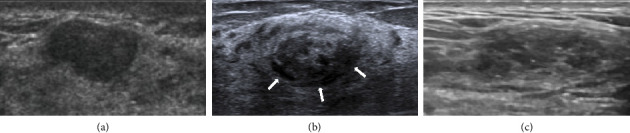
(a) Ultrasound presentation reveals an elliptical, well-defined hypoechoic mass with clear boundaries, (b) cystic hypoechoic areas could be detected (“↑”), and (c) punctate hyperechogenicity was seen within the lesion.
